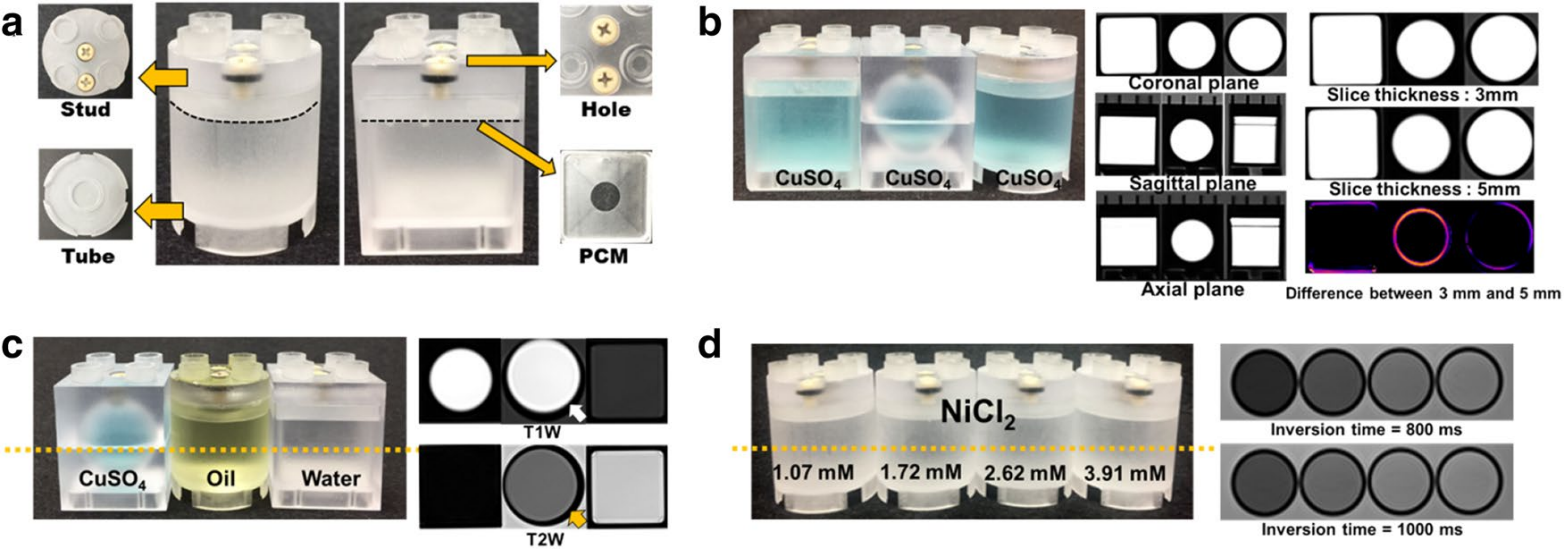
The BM fabrication and imaging characteristics. (a) The BM has LEGO-compatible stud-and-tube mechanical couplings and can be easily combined with LEGO products. The BM includes nut holes through which solutions can be injected and sealing rounded head screws that prevent leakage. Air bubbles that generate artifact on images are separated by a thin PCM attached under the stud coupling part. The cylinder and cubic BM have cylinder and cuboid inner structures. (b) The cubic, sphere, and cylinder BM containing CuSO_4_ and corresponding T1W images with different slice directions of BMs. The spherical BM images have circular cross-section in any direction. The cylinder and cubic BMs show different cross-section images according to the slice directions. Coronal T1W image with different slice thicknesses. The sphere type BM was more affected by slice thickness parameter than the cubic and cylinder type BM due to the edge curvature in-plane. (c) The various inner shapes of BM containing CuSO_4_, oil, and water and corresponding T1W and T2W images. The BM containing CuSO_4_ with the shortest T1 value has high intensity than oil and water BM on T1W images. Water BM with the longest T2 value has high intensity than CuSO_4_ and oil BM on T2W. The white arrow on T1W and the black arrow on T2W shows a chemical shift artifact manifested in the frequency-encoding direction. Image contrast depends not only on the aqueous solution characteristics, but also on the molar concentration of the substance. (d) NiCl_2_ solutions with various concentrations of 1.07, 1.72, 2.62, and 3.91 mM were injected in four cylindrical BMs. The axial MR images along the yellow dotted line are shown in the right. The signal intensities inside the BMs were lineally correlated to the NiCl_2_ concentrations.
